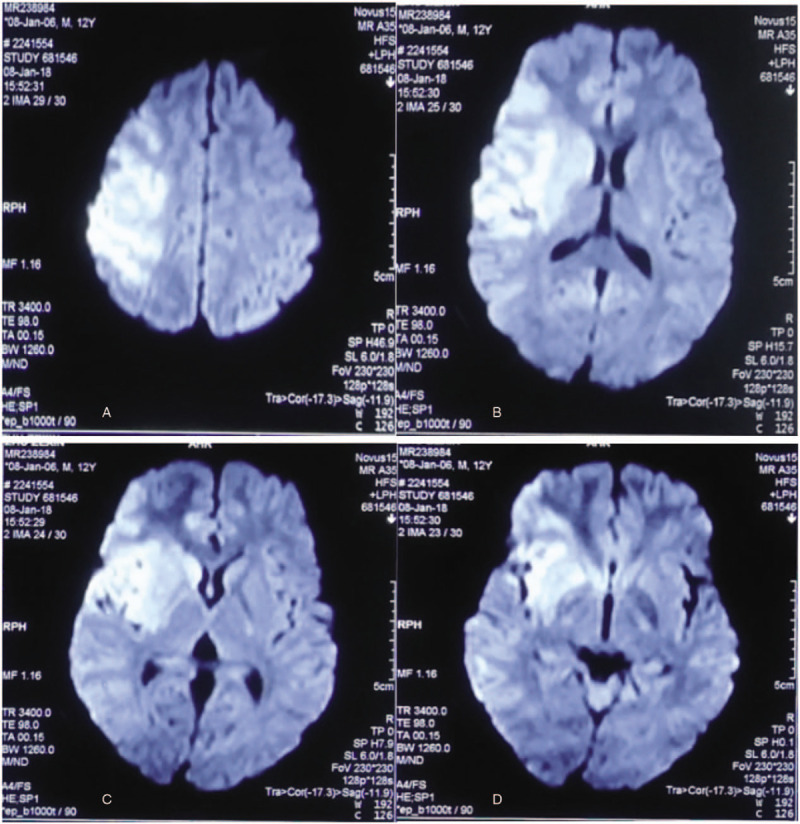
Brain magnetic resonance imaging (MRI; January 8, 2018). Acute cerebral infarction in the right parietal (A), temporal (B,C), insular (B,C), and frontal lobes (D).
